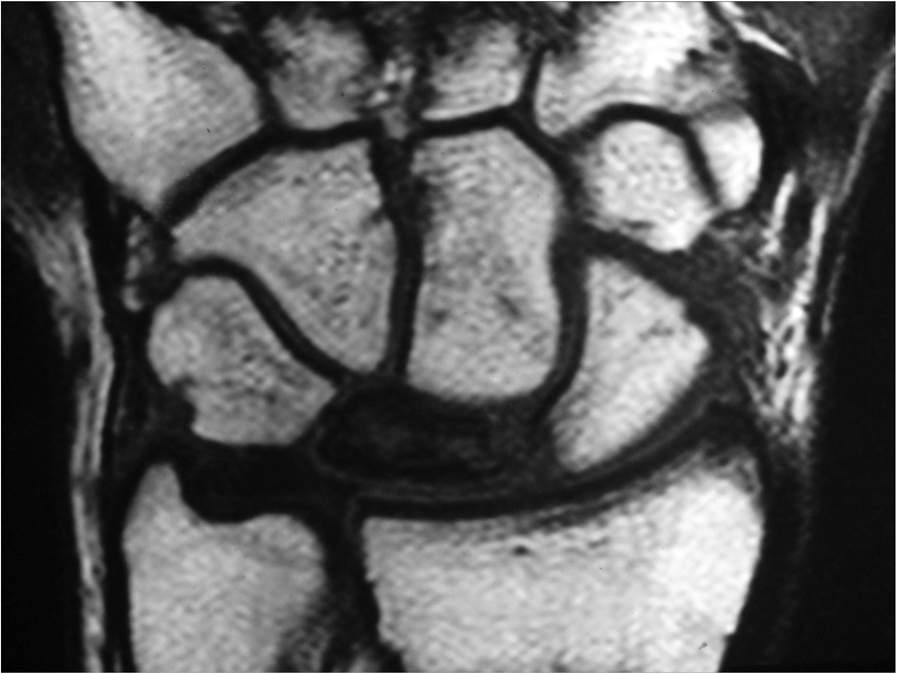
T1-weighted MRI showing decreased signal intensity in the lunate bone.
